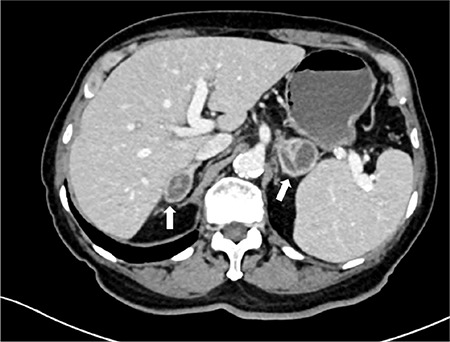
Computed tomography upper abdomen venous phase showing 2.0×2.9 cm mass in the right adrenal gland and 3.7×4.3 cm mass in the left adrenal gland. Both masses (white arrow) show rim enhancement with septation compatible with thin-walled abscess.
